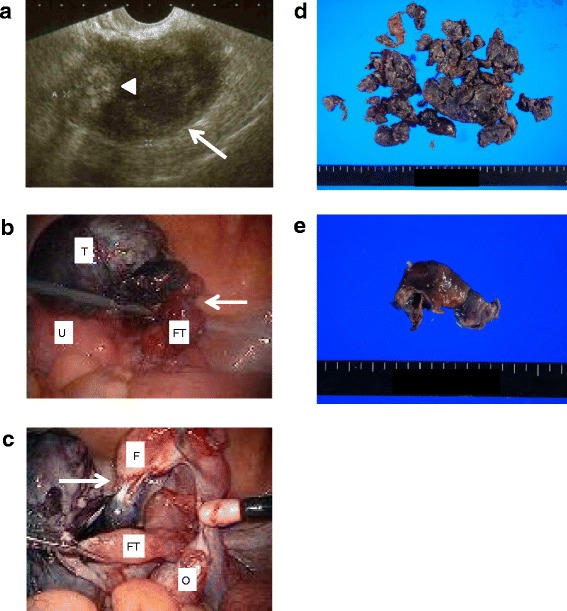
Pre-operative, peri-operative, and macroscopic images in case 1. a: Transvaginal ultrasonography demonstrated a 73 × 47 mm cystic mass (*arrow*) with a partial internal high-echoic lesion (*arrow head*). b: Laparoscopic view of the right fallopian tube (*arrow*). *U* uterus, *FT* right fallopian tube, *T* tumor. c: The area shown in Fig. 1b after detorsion. The tumor can be seen arising from the distal end of the right fimbria (*arrow*). Note the improved color of the right fallopian tube. *F* right fimbria, *FT* right fallopian tube, *O* right ovary. d: Macroscopic image of the excised solid tubal tumor. Contents of the tumor were black and fragmented. e :Macroscopic image of the removed right fallopian tube. The tumor origin site was not clear
